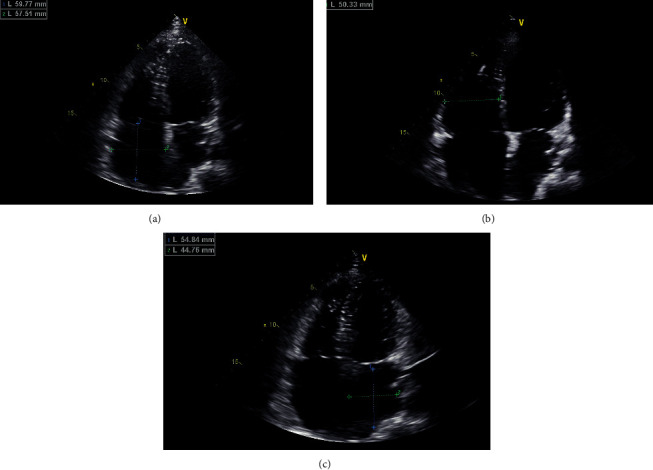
Echocardiography of the proband. (a) Right atrium (59 × 57 mm). (b) Right ventricle (50 mm). (c) Left atrium (54 × 44 mm).
